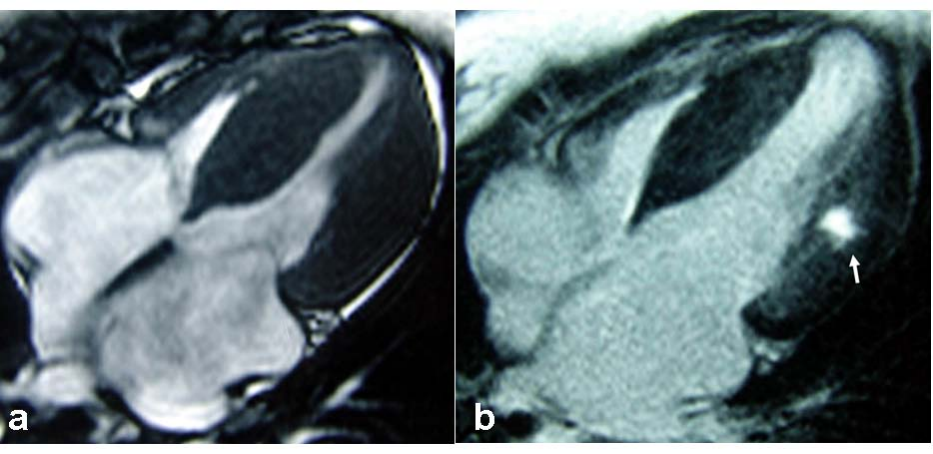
MRI imaging of the case. a A four-chamber view demonstrates symmetrical thickening of interventricular septum and the lateral wall of left ventricle. b A four-chamber view shows fibrosis involving the middle layer of left ventricle lateral wall (arrow).
